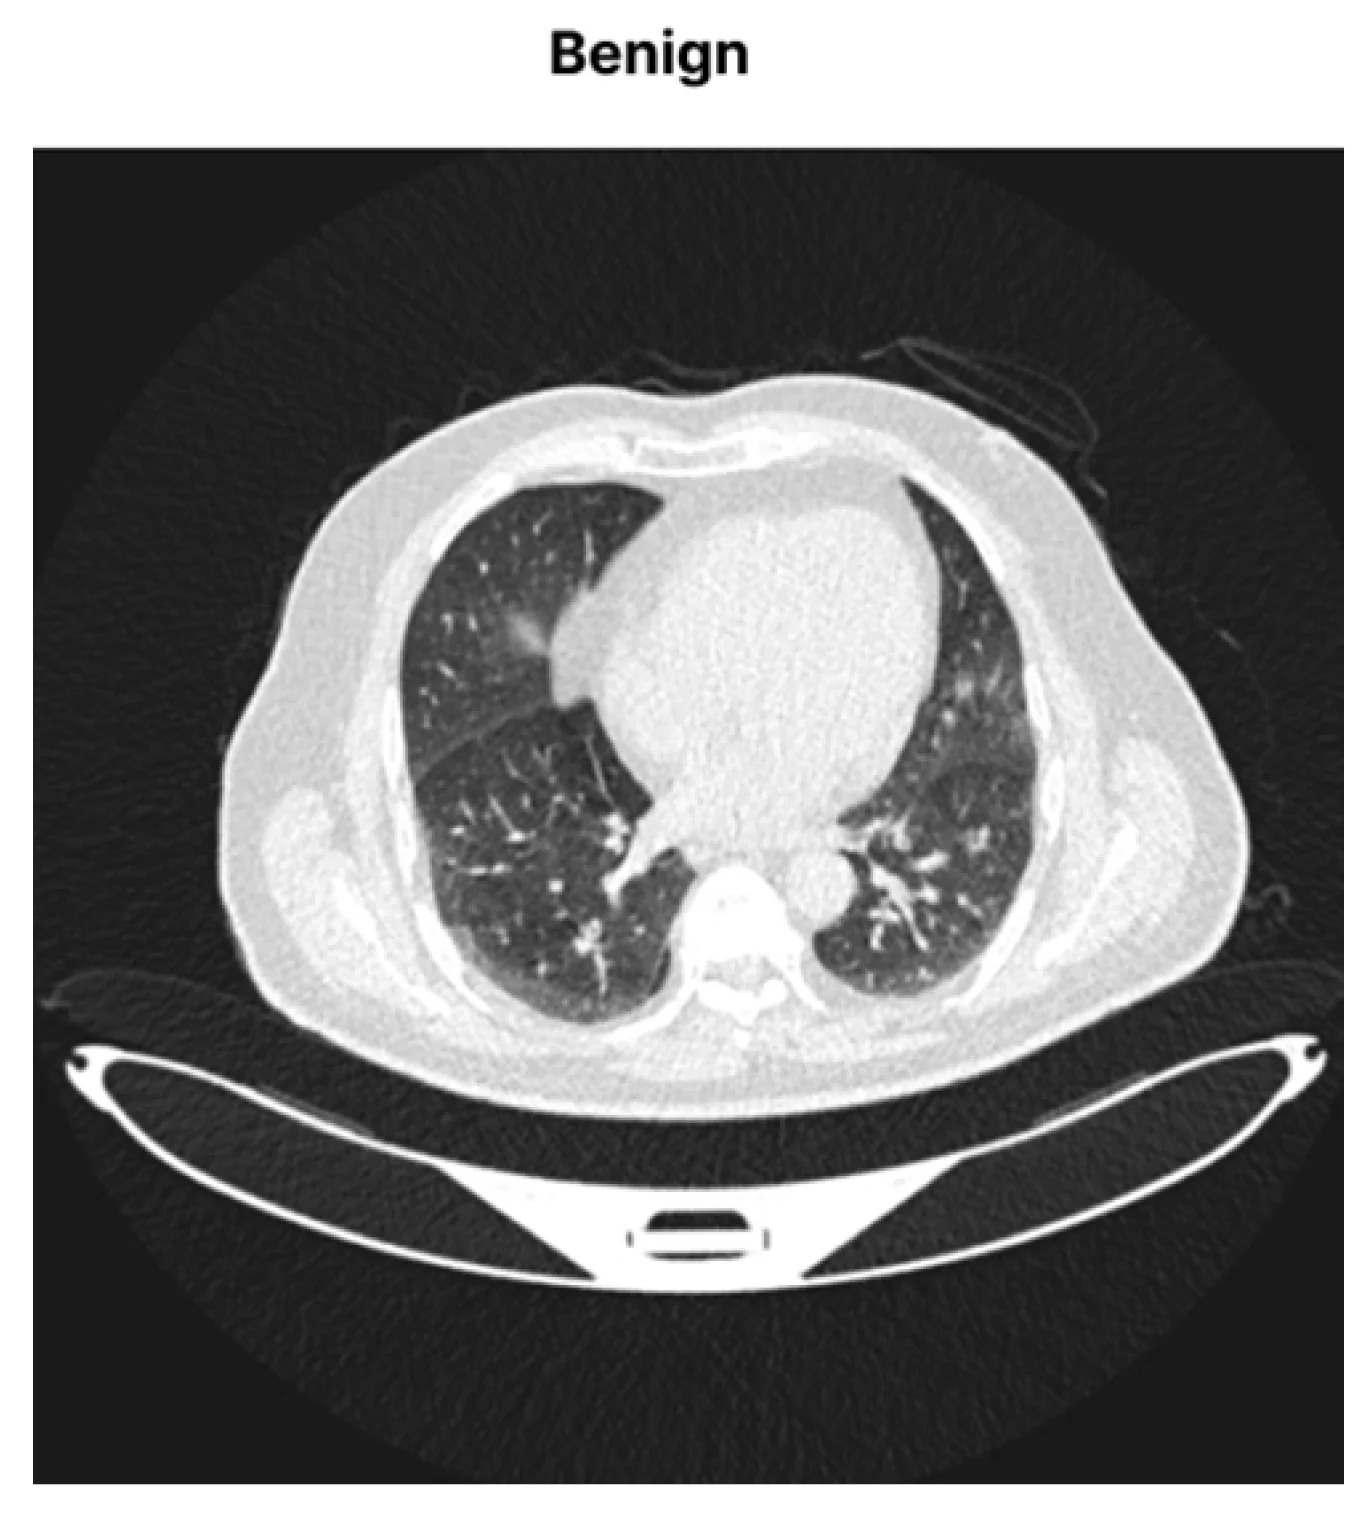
Sample CT scan showing a benign lung nodule.
